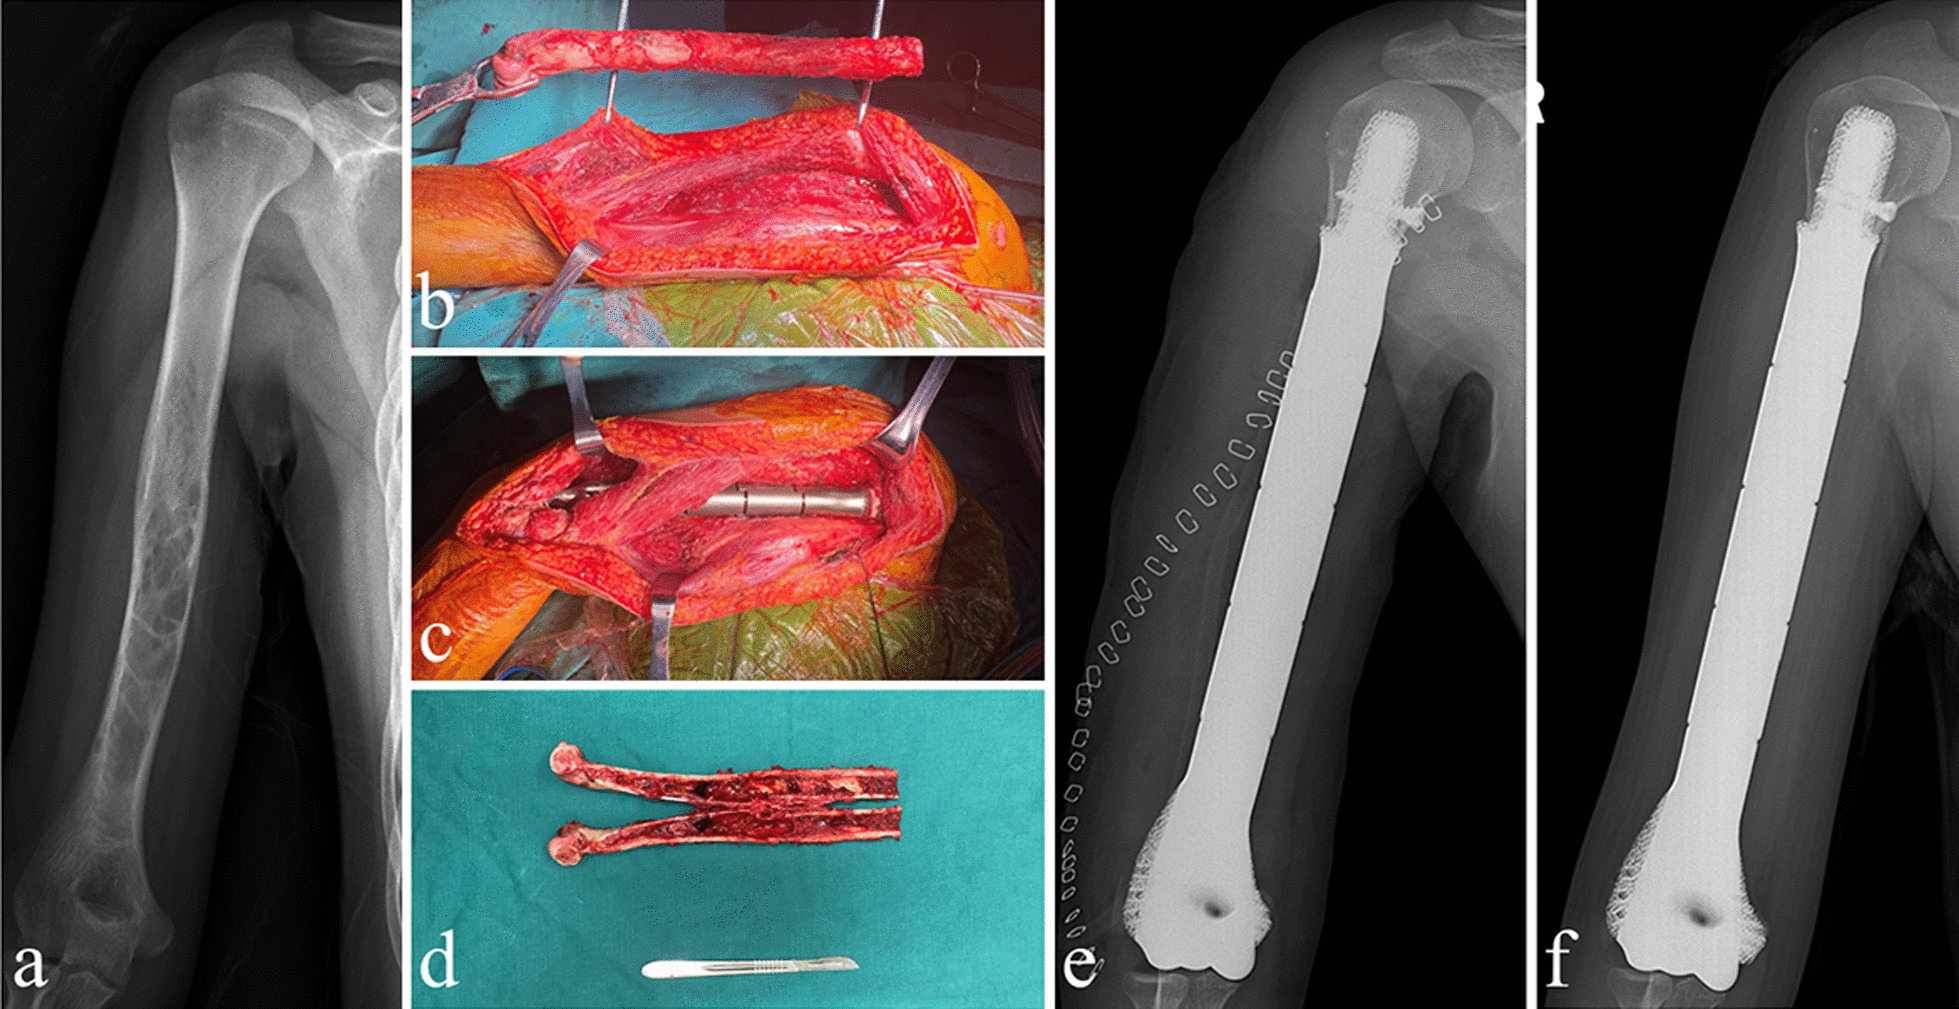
A woman aged 17 years with osteosarcoma underwent reconstruction with a 3DP custom-made short-stemmed endoprosthesis. a Preoperative X-ray; b–d intraoperative pictures; X-ray after surgery immediately and (e) and 12 months (f) after surgery
